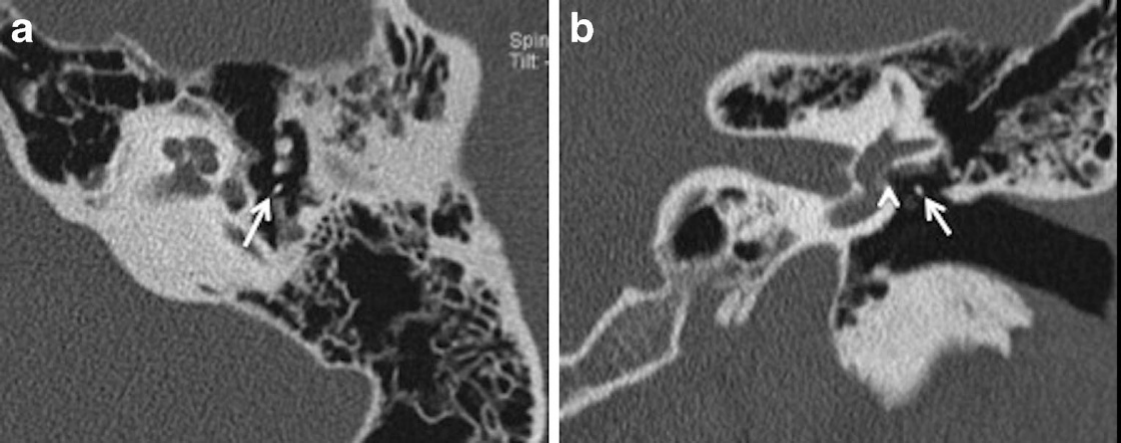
Axial (a) and coronal (b) HRCT images of the left temporal bone in a patient with persistent CHL, post stapedectomy. The stapes prosthesis (*arrow*) is dislocated posteriorly in relation to the oval window (*arrowhead*) with complete loss of contact
